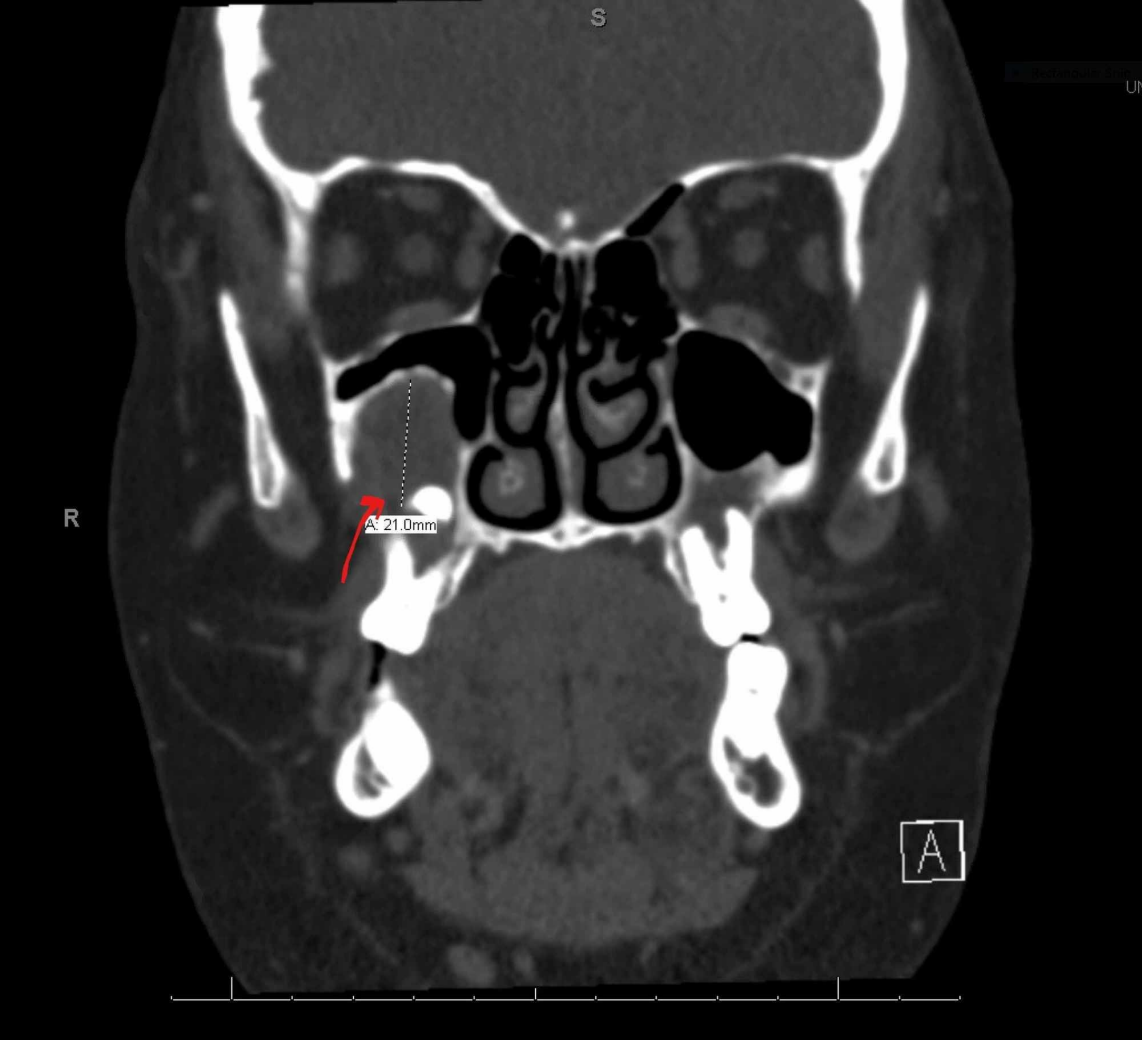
CT maxillofacial and neck showing right maxillary molar (unilocular) dentigerous cyst measuring 2.1 cm x 1.9 cm x 1.6 cm. The red arrow is indicating the right maxillary dentigerous cyst.
